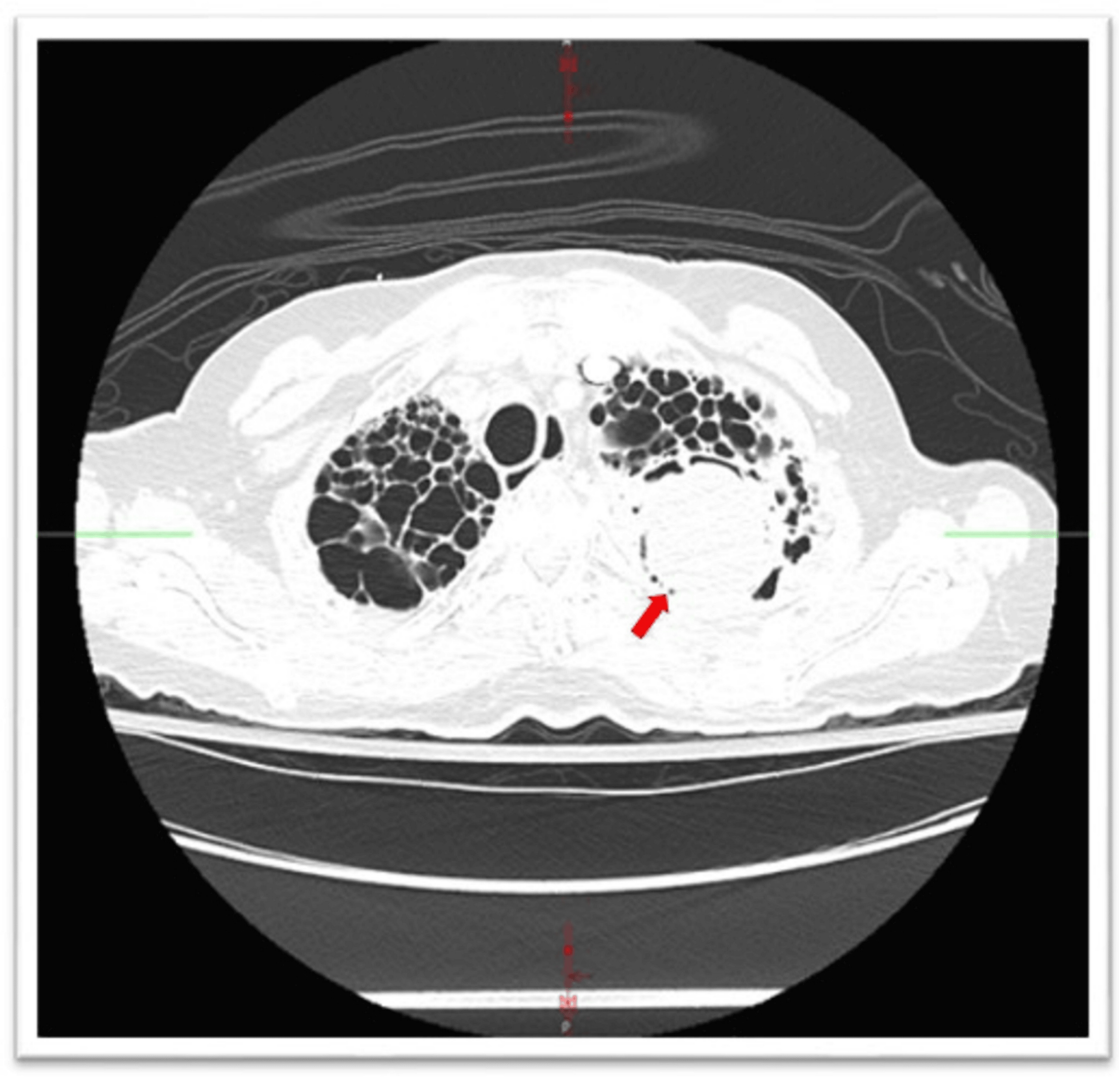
Axial section of the chest CT with contrast showing extensive cystic lesions at bilateral upper lobes and a left upper lobe aspergilloma inside the cavitary lesion (arrow).
